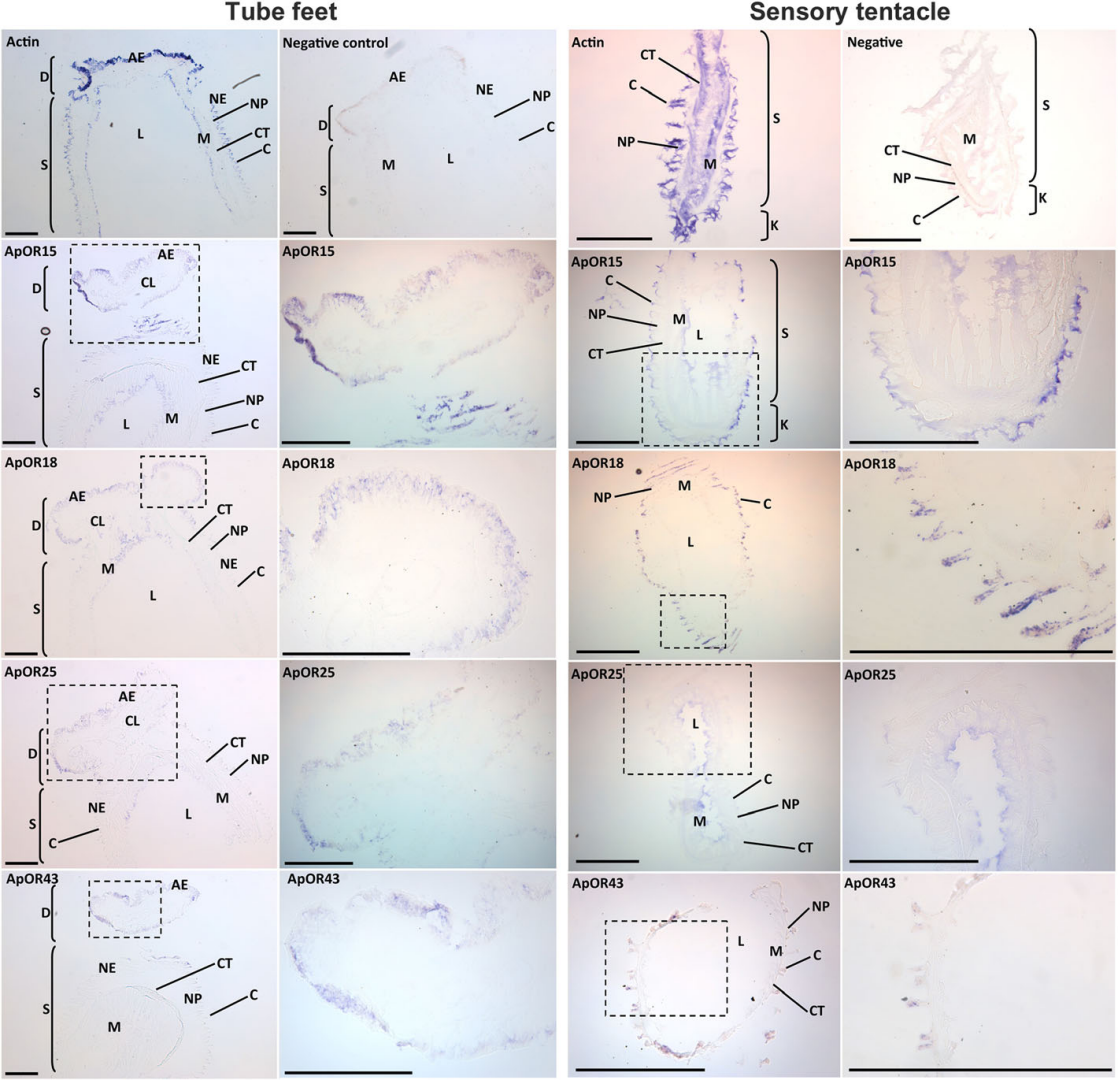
In situ hybridisation of COTS tube feet and sensory tentacles using digoxigenin-labelled antisense RNA probes to show spatial expression of candidate olfactory receptors, ApOR 15, 18, 25, 43, and positive control actin. AE, adhesive epidermis; NE, Non-adhesive epidermis; CL, connective tissue radial laminae; CT, connective tissue layer; D, disc; S, stem; L, water-vascular lumen; M, myomesothelium; NP, nerve plexus; C, cuticle; E, epidermis. Scale bars = 200 μm
